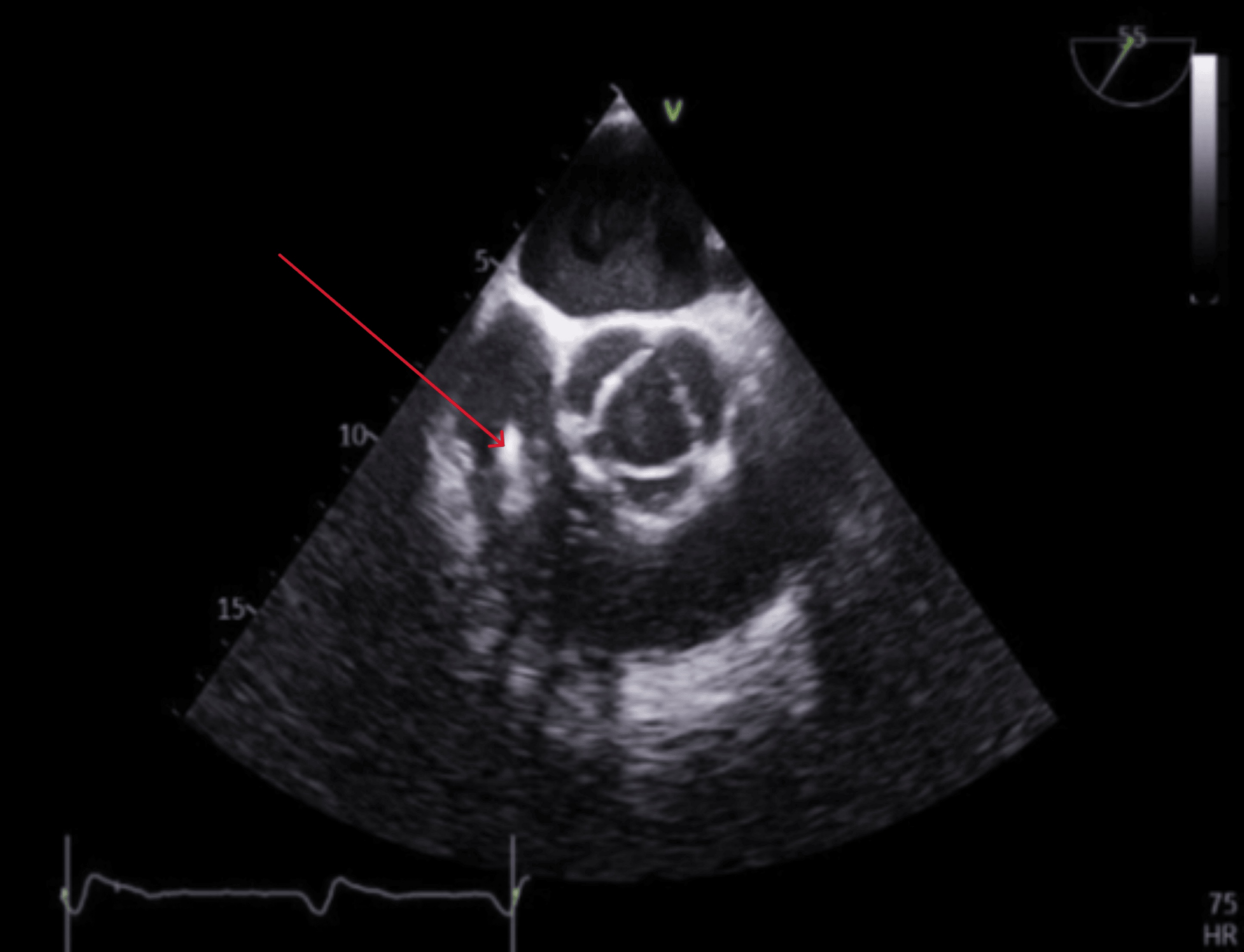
Transoesophageal echocardiogram showing echogenic shadowing of at least 2.5 by 1.3 cm in diameter on the right ventricular pacing lead consistent with vegetation.
